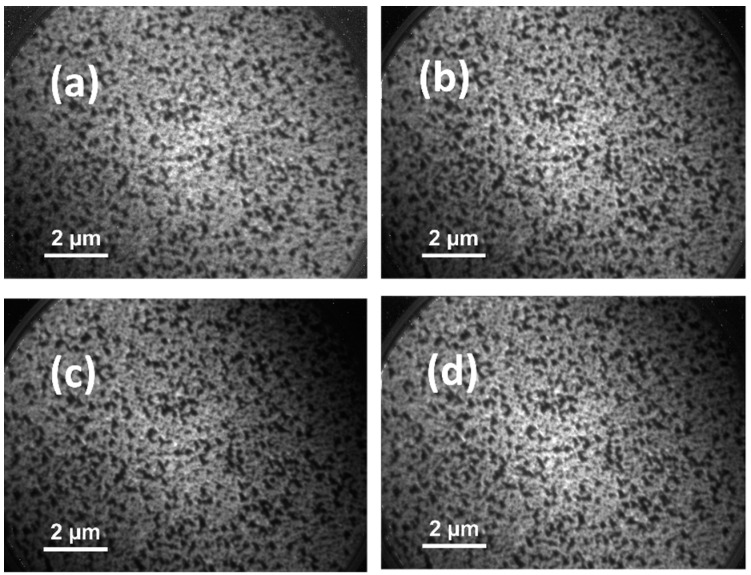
XPEEM images showing chemically selected scans of W 4 f. The images were recorded with a 12.4 µm field of view (FoV), corresponding to the diagonal distance in the images given. The X-ray excitation photon energies is increasing from 75 to 90 from (a–d) with a step size of 5 eV.
